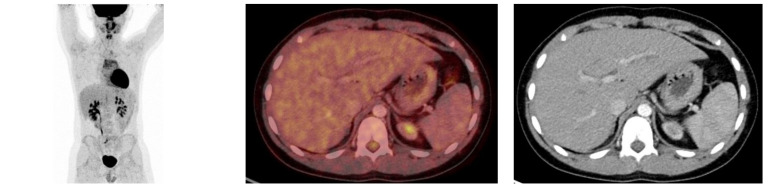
Follow-up: ^18^F-FDG-PET/CT maximal intensity projection (left), transaxial fused PET/CT image (middle) and CT image (right) of the liver where the previously seen lymph nodes in the liver hilum are no longer visible.
